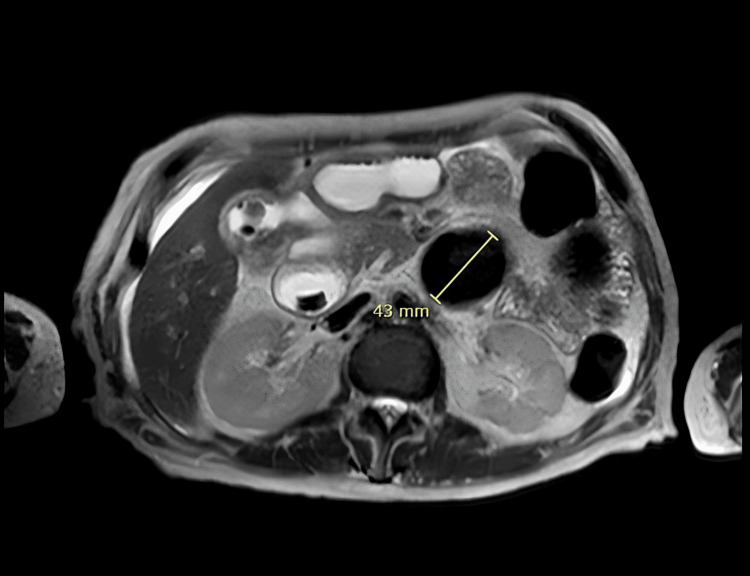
T2-weighted magnetic resonance axial image of a large gallstone, cholelithiasis, and cholecystoduodenal fistula.
